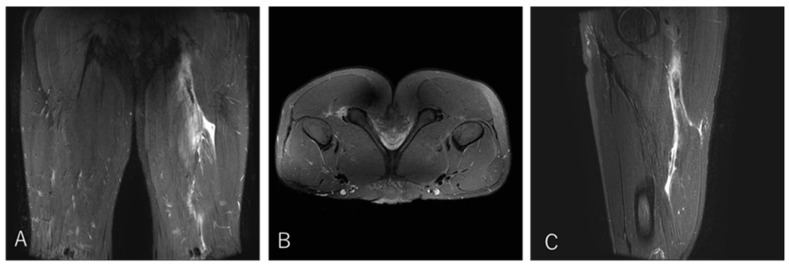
Case 1 MRI images: Conjoint tendon detachment, Type I. (A) (Coronal image): The CT (conjoint tendon) is damaged near the ischial tuberosity, with the haematoma extending to the MTJ. However, the retraction of the ruptured tendon is not severe. (B) (Axial image): prone position, The CT is detached from the ischial tuberosity in zone A. The SM tendon is normal. (C) (Sagittal image): The injury is located proximally to the CT, with the haematoma extending distally into the inter-muscular space.
